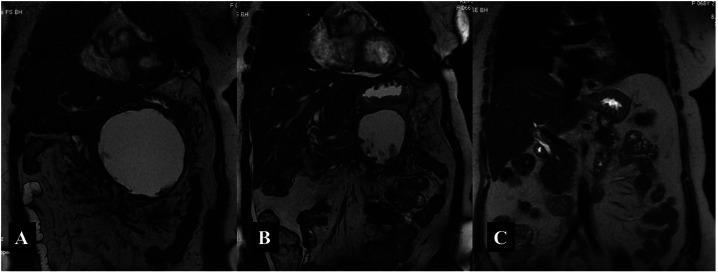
A 66-year old asymptomatic female. A) An 11 cm-sized WON in the midline of the abdomen. B) Reduction of WON size to 7 cm on MRI after 5 months. C) Complete resolution of WON observed on MRI obtained after 9 months.
